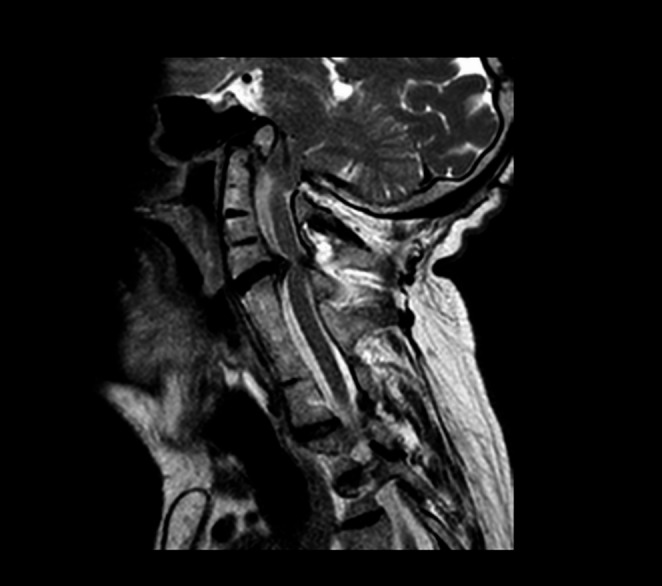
MRI scan of cervical spine. T2-weighted, sagittal view MRI of the cervical spine showed a complex cervical-occipital malformation. The fusion of multiple cervical vertebrae, associated with spinal canal stenosis and C4-C5 disc degeneration, was also evident
